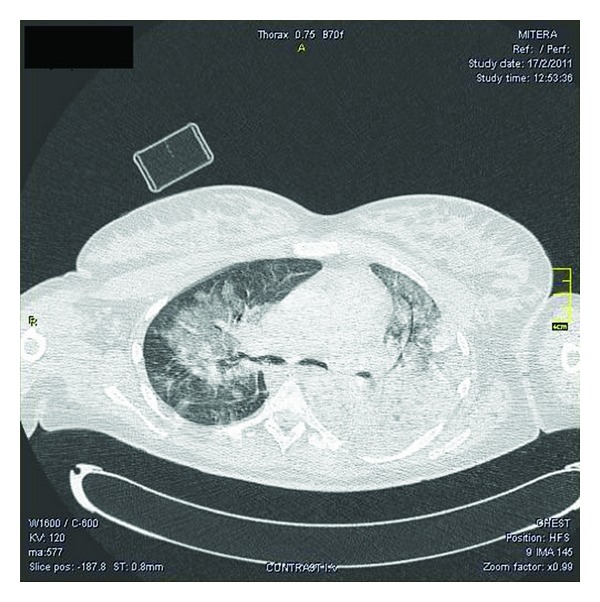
Chest CT confirming bilateral basilar pulmonary consolidation, consistent with pneumonic infiltrates.
